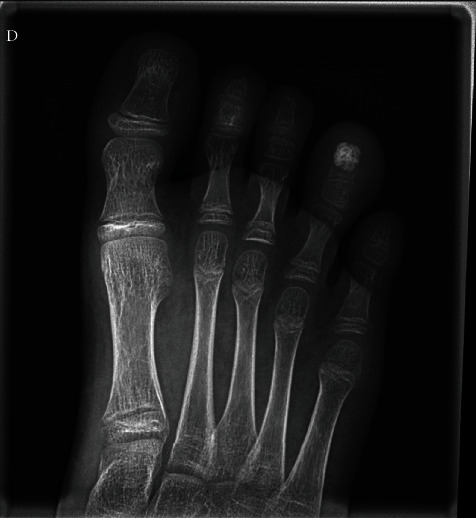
X-ray demonstrating the large nidus and the preservation of the proximal epiphysis. The important swelling of the fourth toe is well demonstrated.
